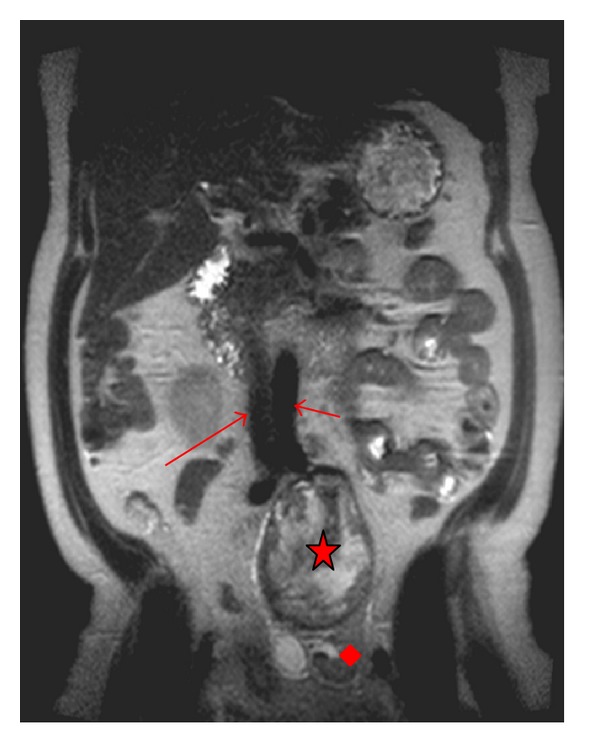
T2-weighted MRI image (coronal view) showing orientation of mass (star) to major vessels. Normal kidney (diamond). Aorta (short arrow). IVC (long arrow). Note that aortic bifurcation occurs at level of superior pole of ectopic left kidney.
